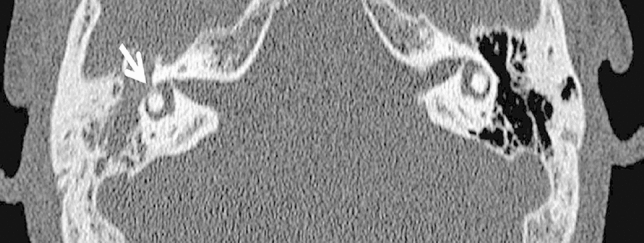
Preoperative CT scan of the temporal bone from one of the patients in the cohort showing erosion of the lateral semicircular canal
